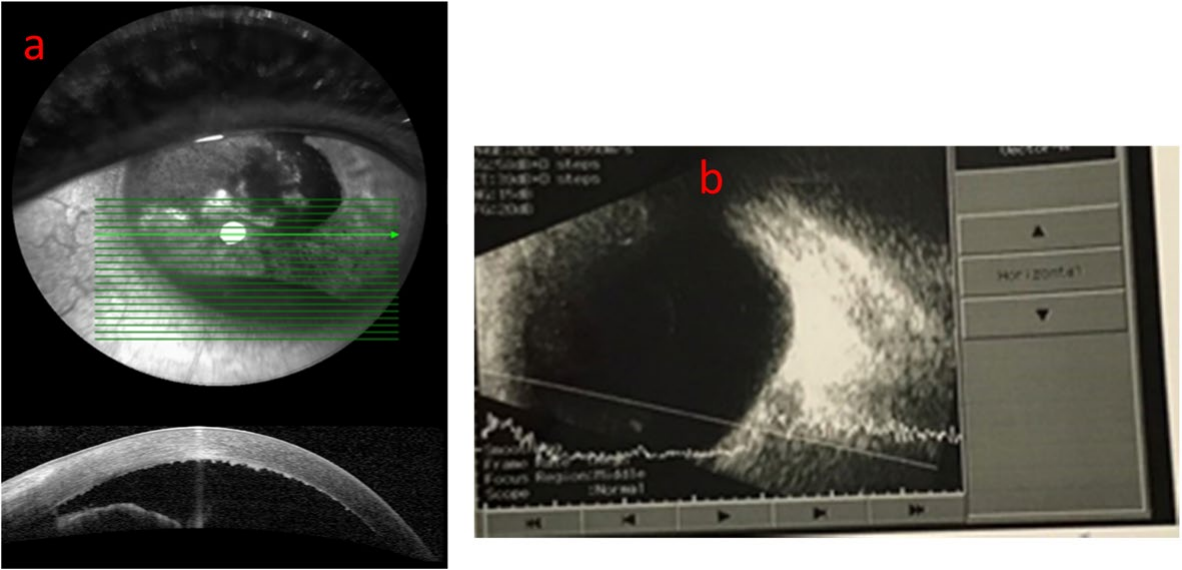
a Anterior segment OCT shows thick hyper-reflective material on the corneal endothelium, elevation and hyper-reflectivity of the iris surface with a beneath hypo-reflectivity. b B scan ultrasonography of the right eye shows no vitreous involvement despite severe hypopyon anterior uveitis precluding fundus exam at first presentation
